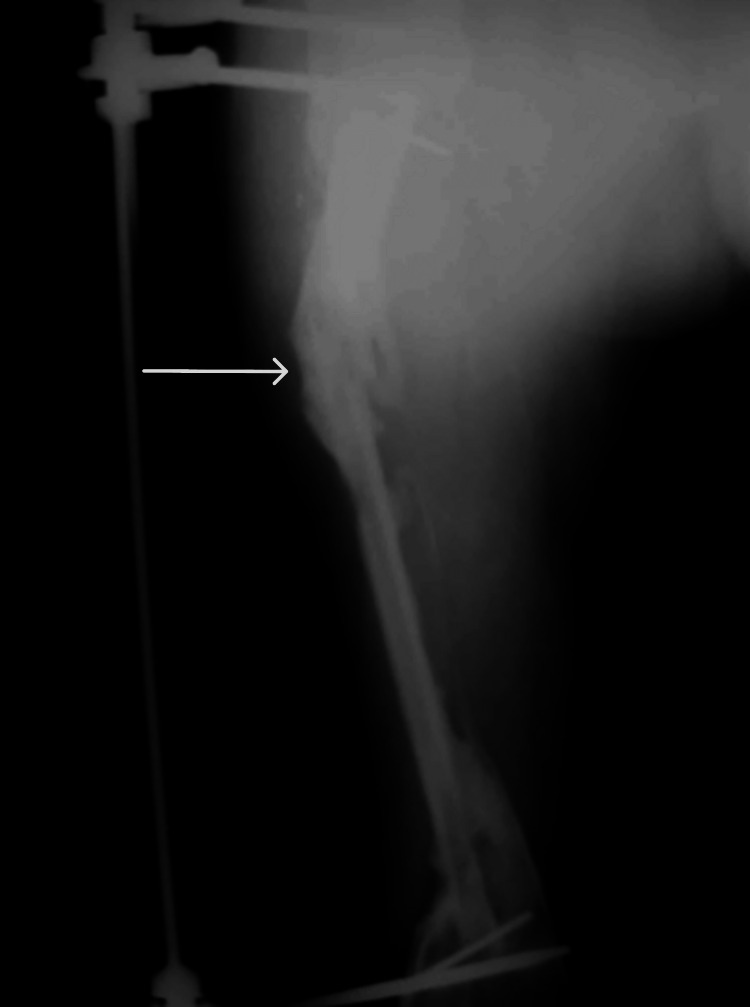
X-ray image 4 months postsurgery showing an upper fibular fracture. Upper fibular fracture is indicated by the arrow.
